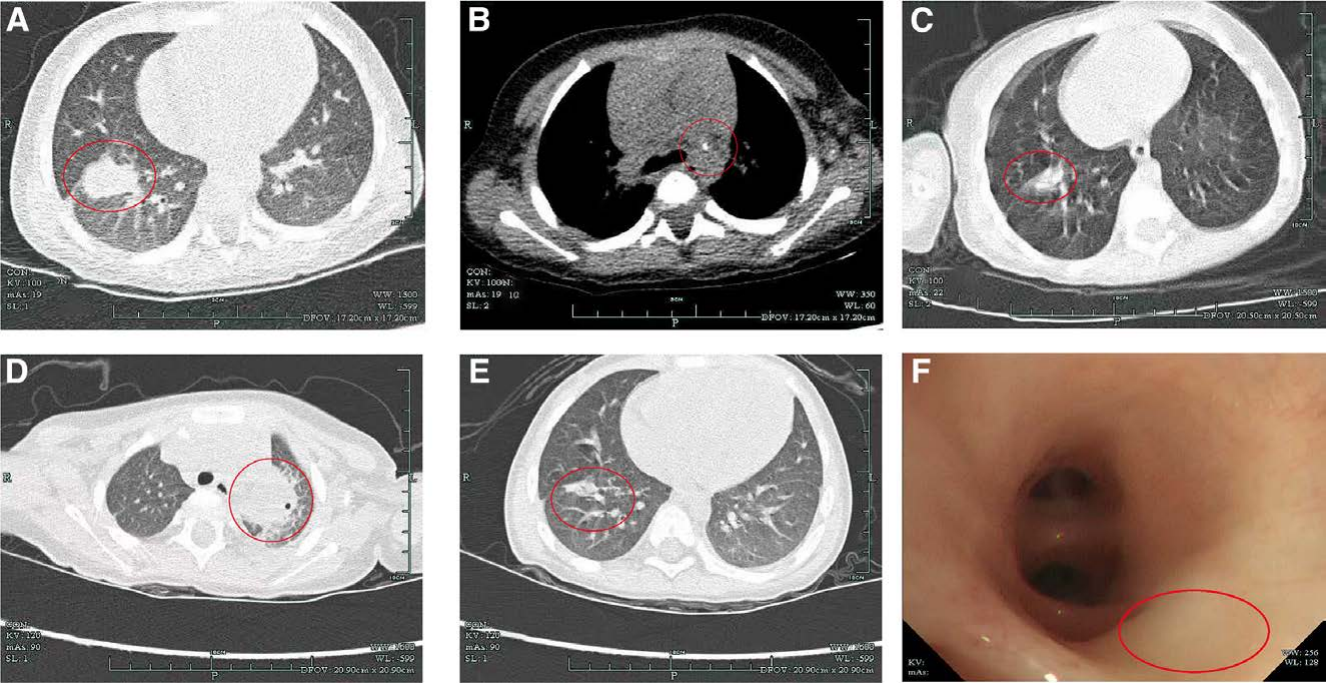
Serial chest computed tomography (CT) and bronchoscopy findings of the patient. (A, B) Chest CT image obtained on August 23, 2022, showing initial pulmonary lesions. (C) Follow-up CT scan on October 17, 2022, indicating disease regression. (D, E) CT scan on July 31, 2023, demonstrating the emergence of new lesions. (F) Bronchoscopy image taken on October 27, 2022, showed mild mucosal swelling in the anterior basal segment of the right lower lobe, revealing airway abnormalities consistent with the CT findings. CT = computed tomography.
